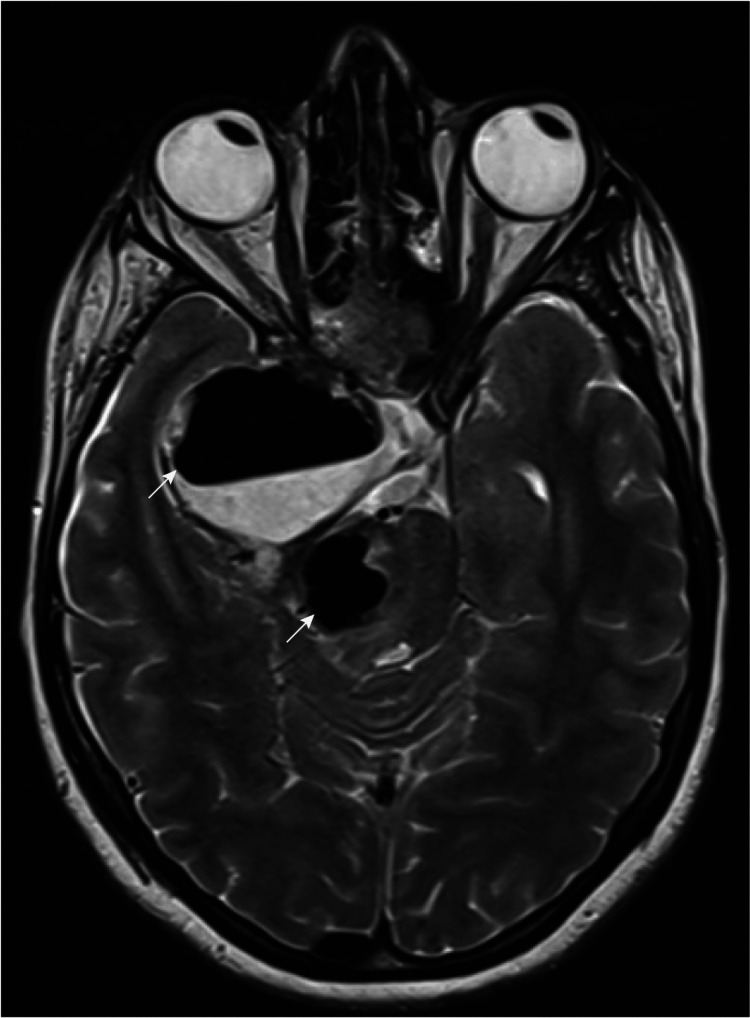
MRI imaging demonstrating localized pneumocephalus within the tumor cavity
